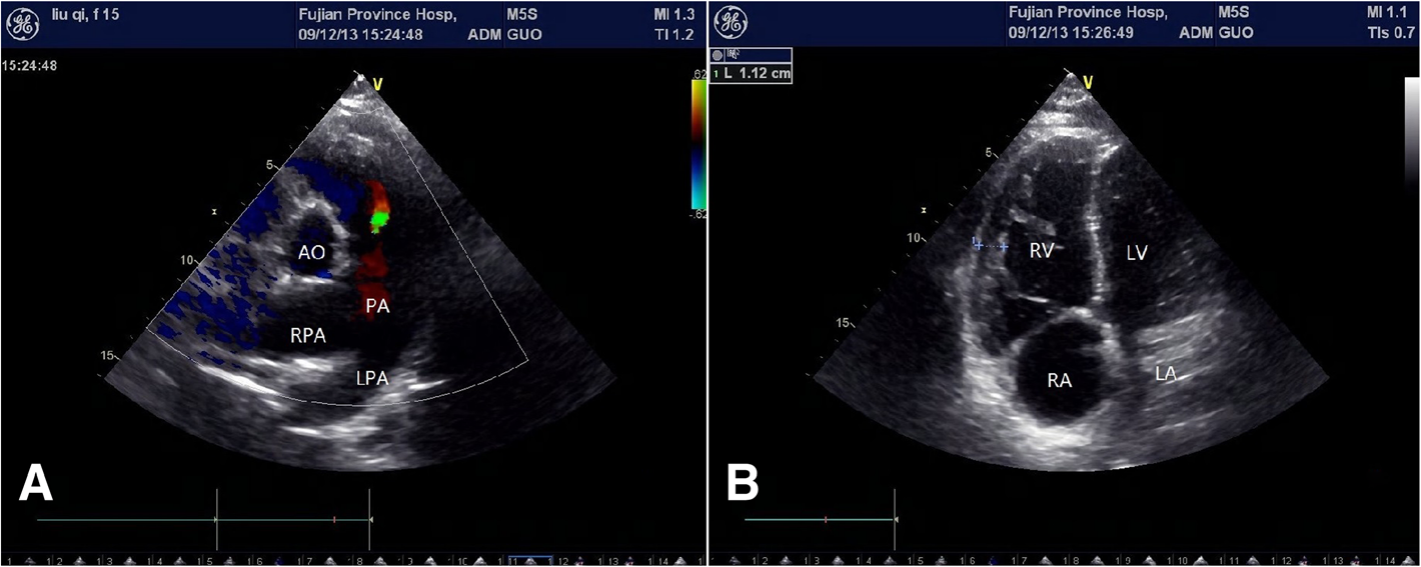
Echocardiography shows an enlargement of the PA and its branches (a). Both RA and RV are significantly dilated, and the RV wall thickness is increased (b). PA: pulmonary artery; RPA: right pulmonary artery; LPA: left pulmonary artery; AO: aorta; RA: right atrium; RV: right ventricle; LA: left atrium; LV: left ventricle
